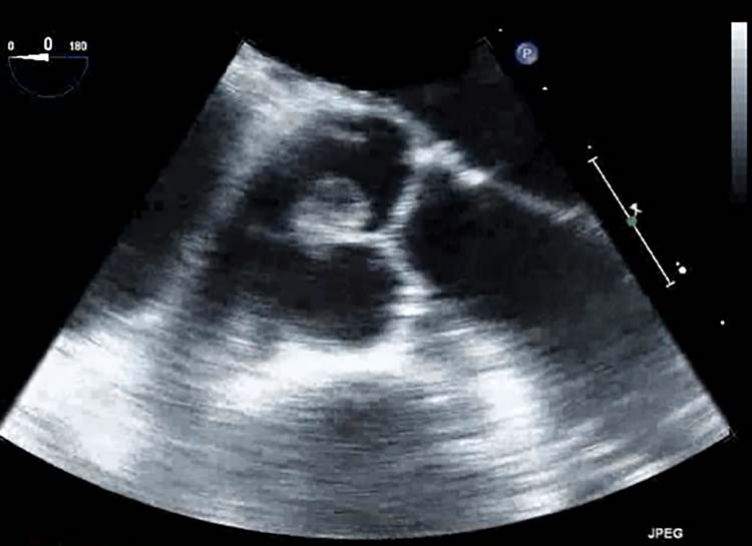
Papillary fibroelastoma seen on trans-esophageal echocardiogram as a pedunculated mass on the aortic valve measuring 16 × 8 mm.
